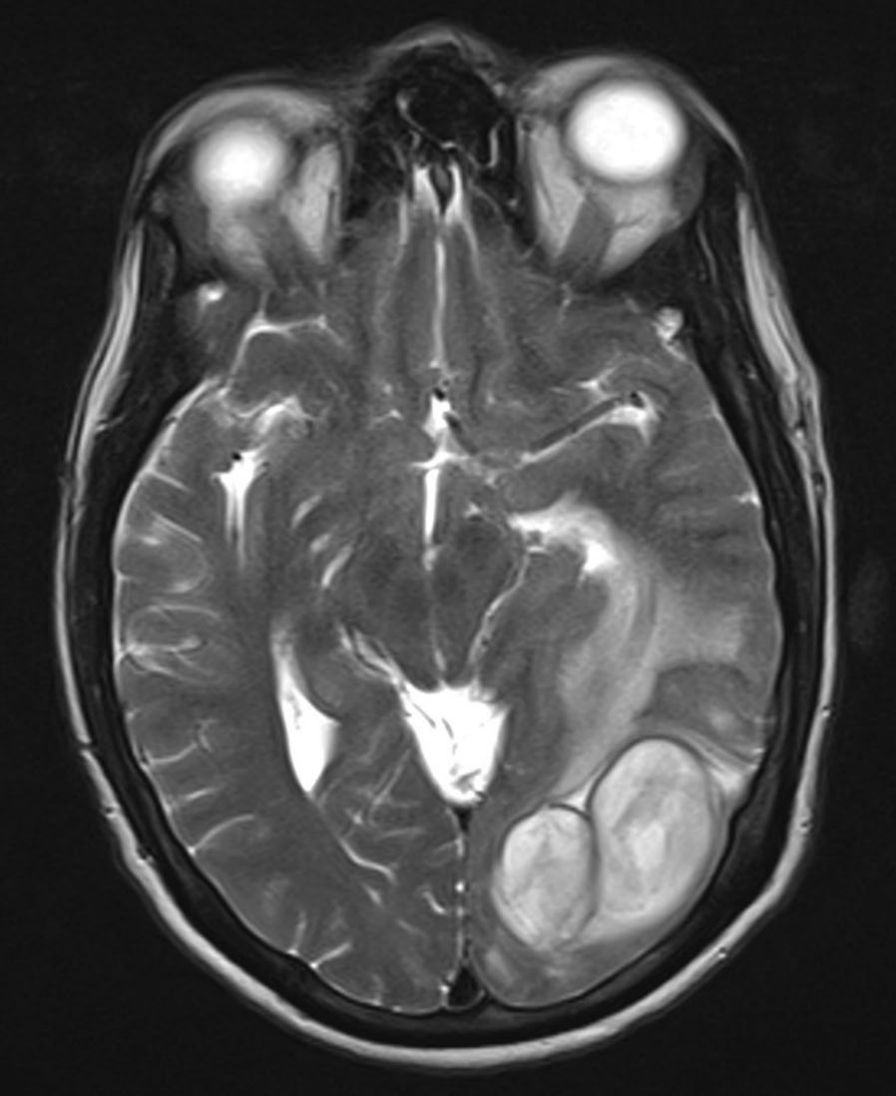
Brain MRI. MRI scan of the brain showing two closely related cerebral abscesses in the left occipital region and resulting peri-lesional edema and the mid-line shift
